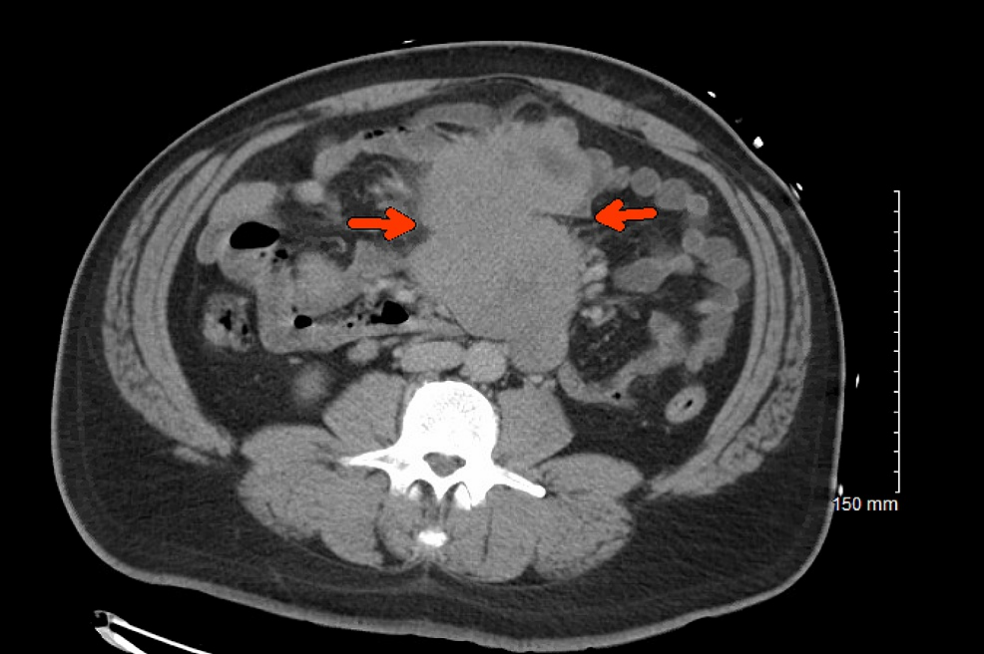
Computed tomography scan showing a large necrotic mass (arrows) along the central mesentery.
